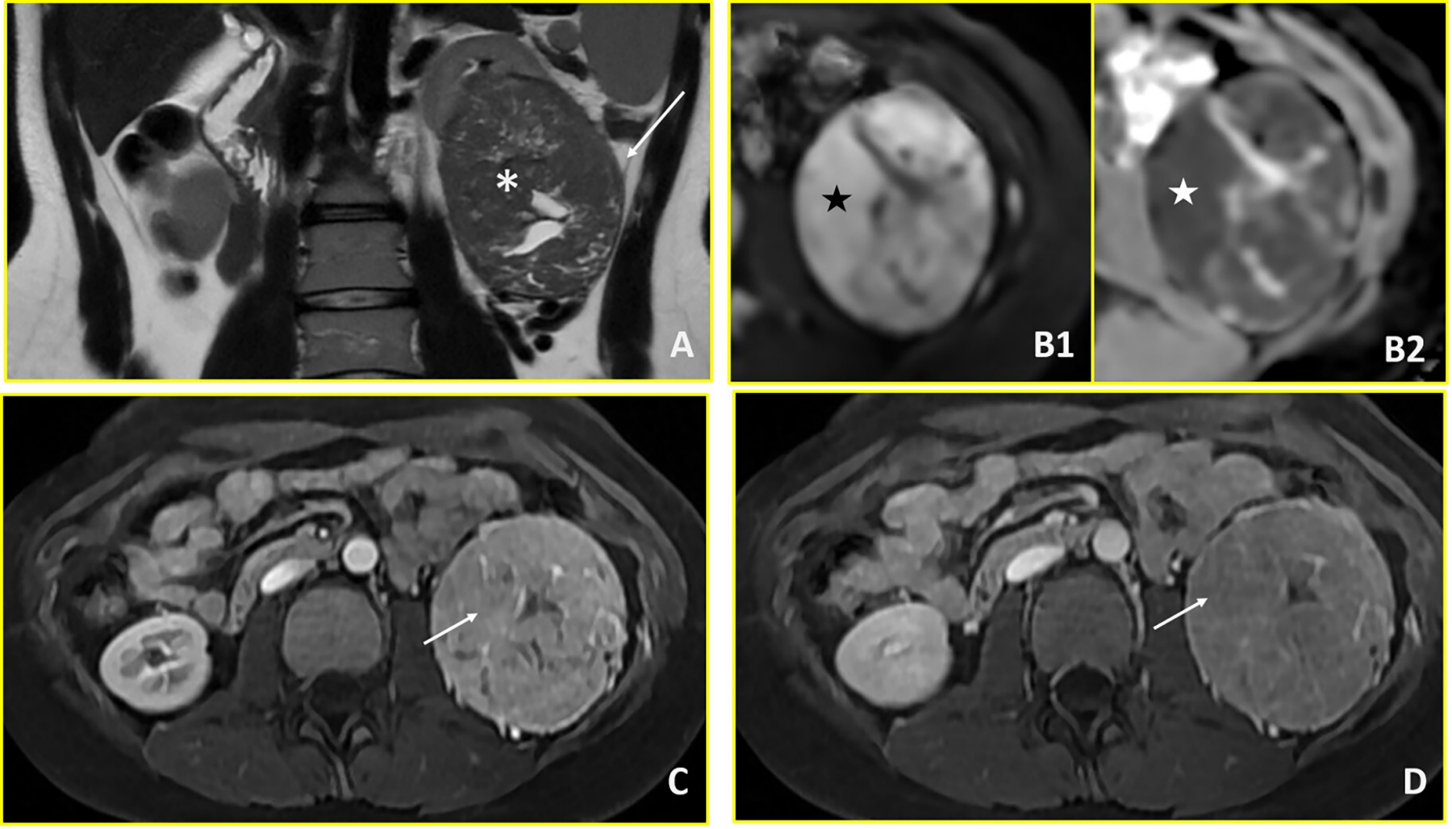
Renal cell carcinoma (Case 2). Multiparametric MRI demonstrates a large left renal mass with imaging features characteristic of renal cell carcinoma. (A) T2-weighted axial image shows a hypointense mass in the left kidney (asterisk) with extension into the perirenal fat (arrow). (B1, B2) Diffusion-weighted imaging reveals restricted diffusion, characterized by hyperintensity on high b-value images (b = 800 s/mm^2^; black star in B1) and corresponding hypointensity on the apparent diffusion coefficient (ADC) map (white star in B2). (C, D) Dynamic contrast-enhanced imaging demonstrates avid arterial-phase enhancement (arrow in C) with subsequent washout on venous-phase imaging (arrow in D), consistent with the hypervascular pattern typical of renal cell carcinoma.
